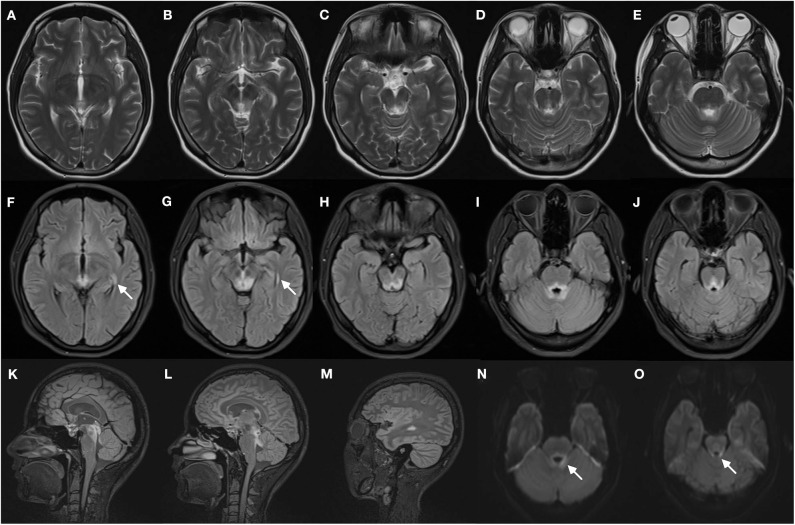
Brain magnetic resonance images of the patient. T2-weighted images (A–E) demonstrate bilateral symmetrical hyperintense lesions in periaqueductal regions and around the fourth ventricle, which include superior colliculus (A), ventral tegmental area (B), inferior colliculus (C), and superior cerebellar peduncle (D,E). The abnormal regions revealed more obvious hyperintensity signal on corresponding FLAIR images (F–J), increased signal in the lateral ventricles was found (F,G, arrows). The FLAIR sagittal slice showed apparent hyperintense signal in the periaqueductal gray region (K) and surrounding the fourth ventricle (L), and lesions in the lateral ventricles (M). DWI images also manifest restricted water diffusion adjacent to the fourth ventricle (N,O, arrows).
